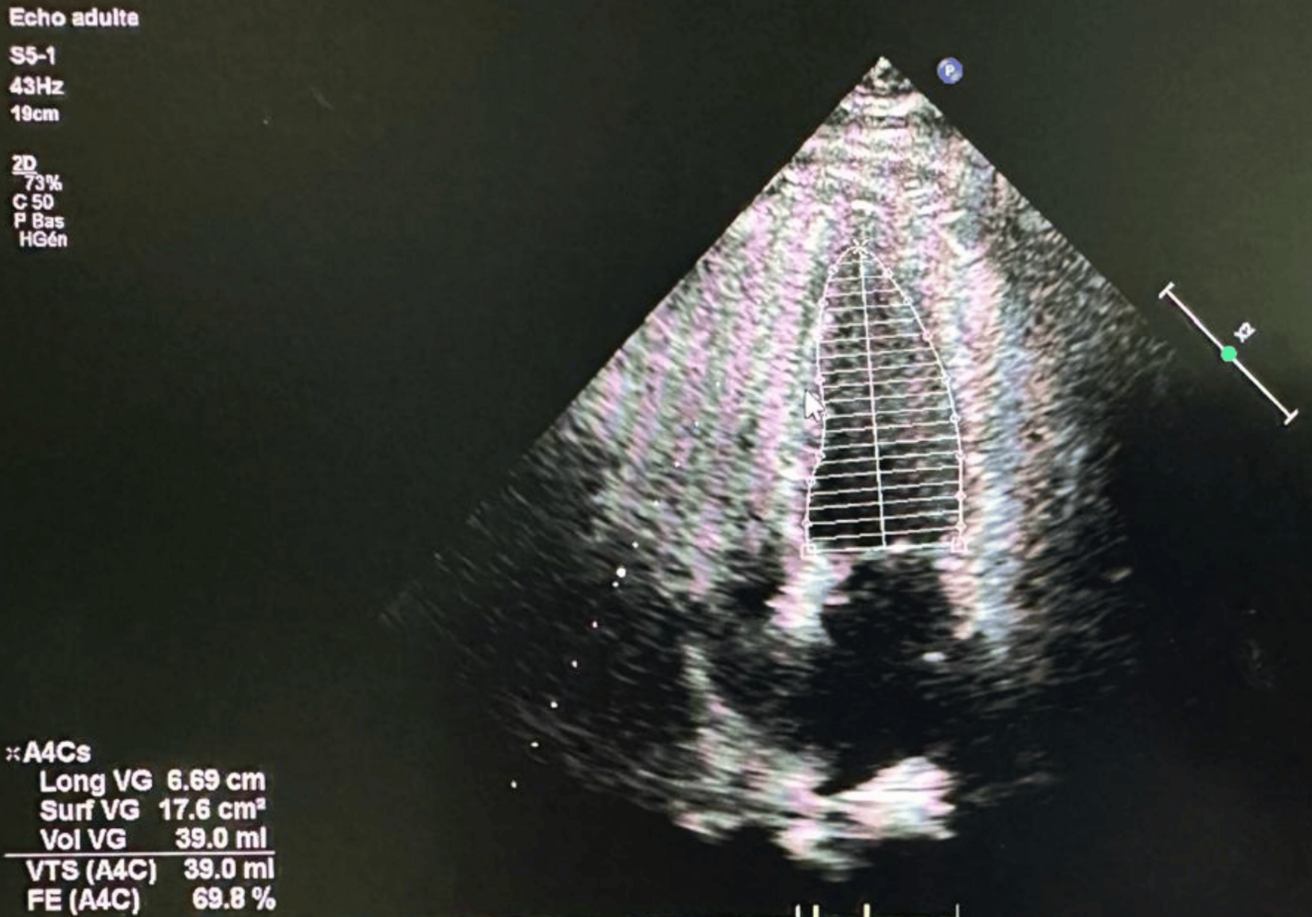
Transthoracic echocardiographic apical four-chamber view showing preserved left ventricular systolic function.
